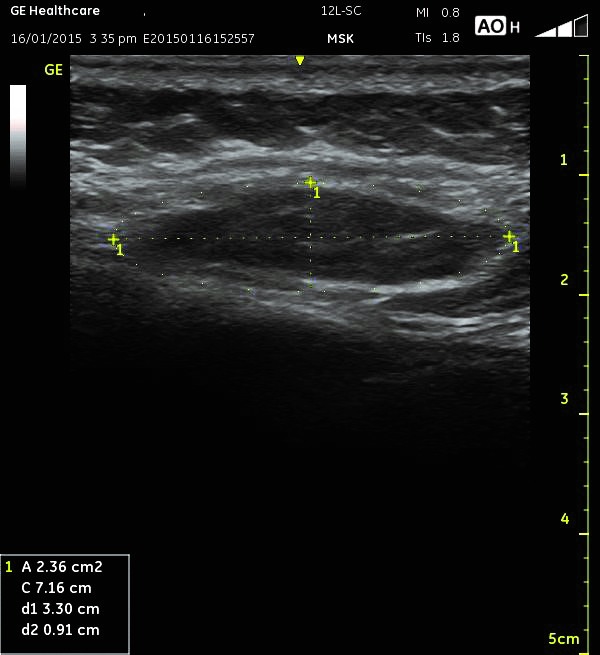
Ultrasound examination of a trigger point
